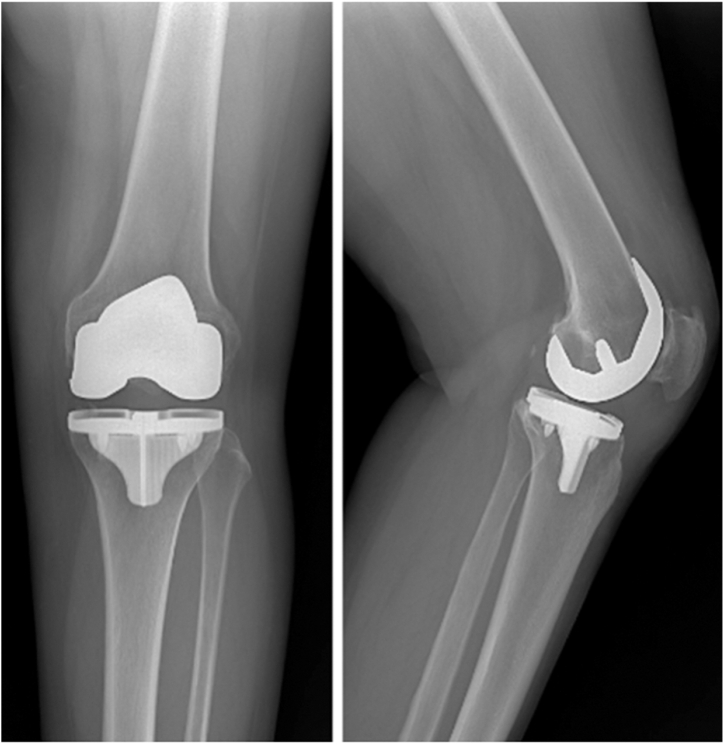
Anterior-posterior and lateral X-rays of the left TKA revision 6 weeks postoperatively.
